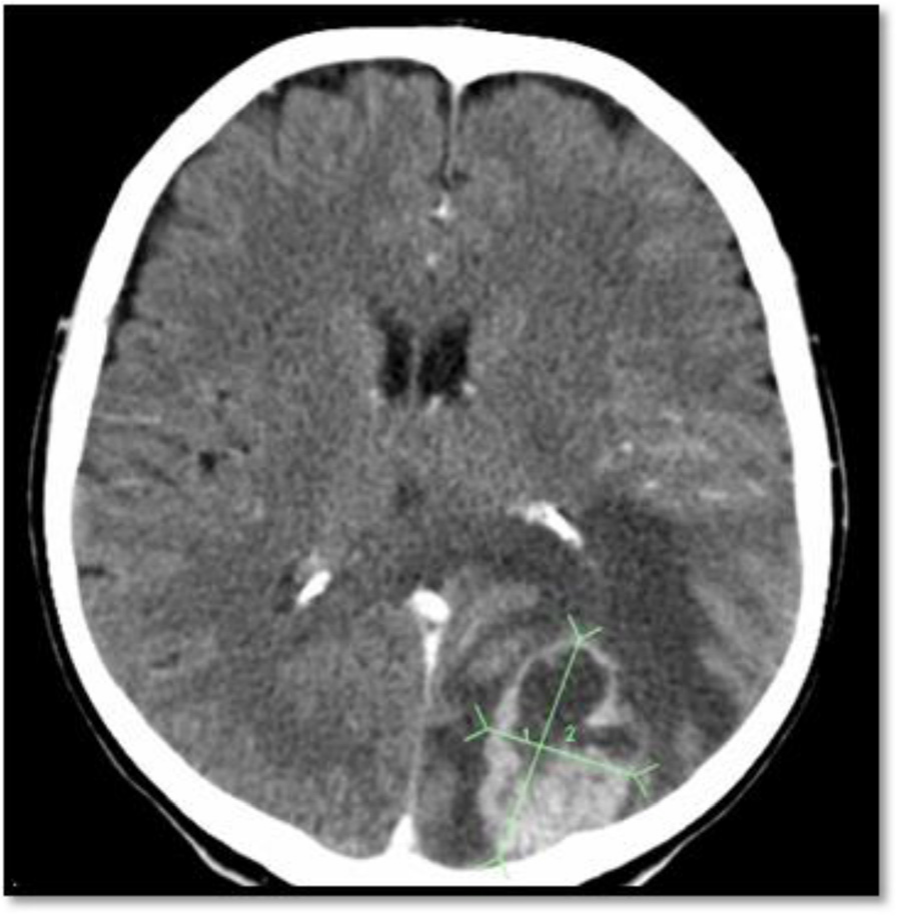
CT scan of the brain showing a solitary metastasis, 5x4 cm, in the right occipital lobe, with some extension to the parietal lobe. Note the heterogeneic appearance of the metastasis and the surrounding brain edema
